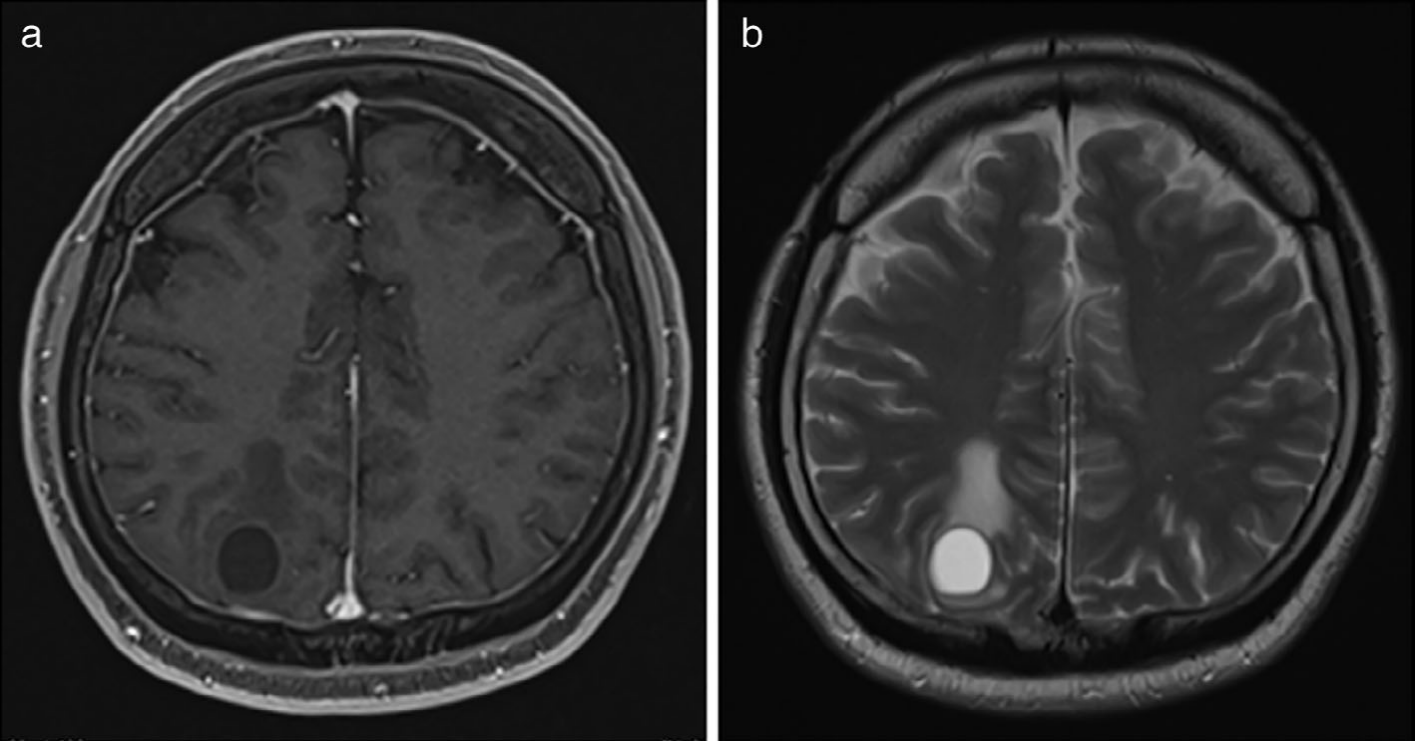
Magnetic resonance imaging (MRI) of brain cavernous hemangiomas mimicking radiation‐induced necrosis. The lesion was hypointense on (a) T1‐weighted image and hyperintense on (b) T2‐weighted image.
